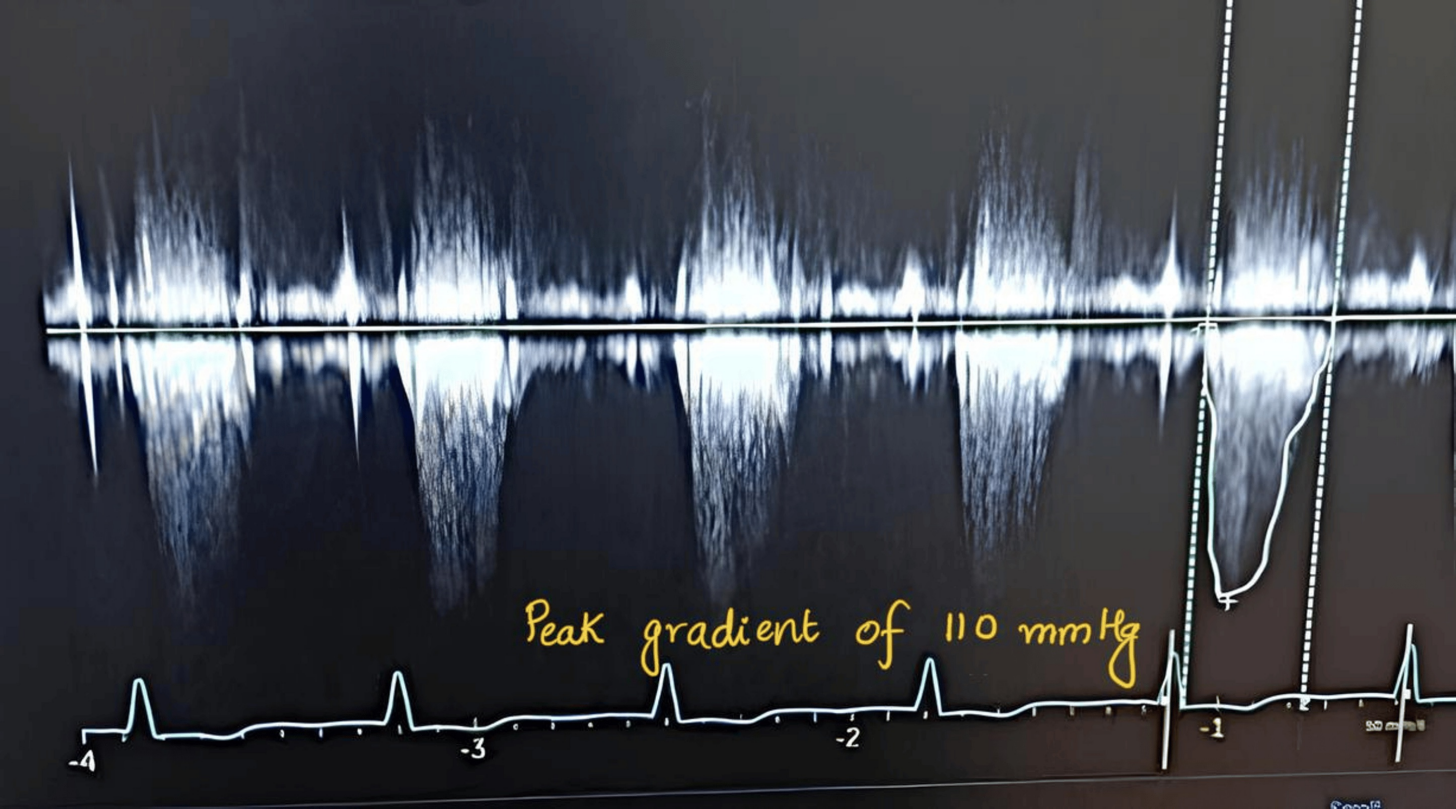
Doppler image displaying LVOT gradient LVOT, left ventricular outflow tract
